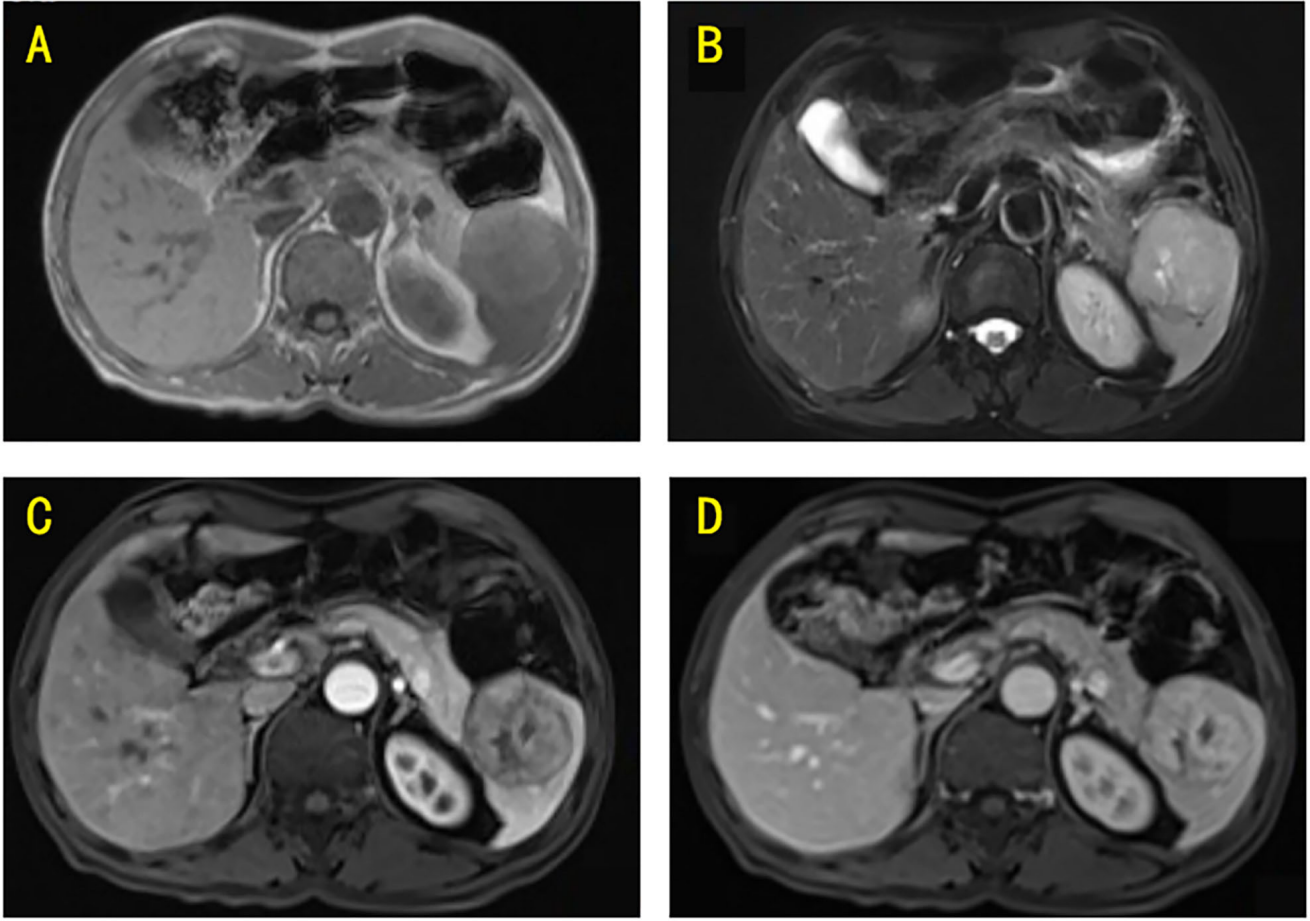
(A) T1-weighted MRI revealed a mass located within the spleen, which demonstrated a slightly hyperintense signal. (B) T2-weighted MRI showed that the mass presented a slightly hyperintense signal. (C) Post-contrast MRI (arterial phase) revealed homogeneous enhancement of the mass. (D) Post-contrast MRI (portal venous phase) showed further enhancement of the mass.
